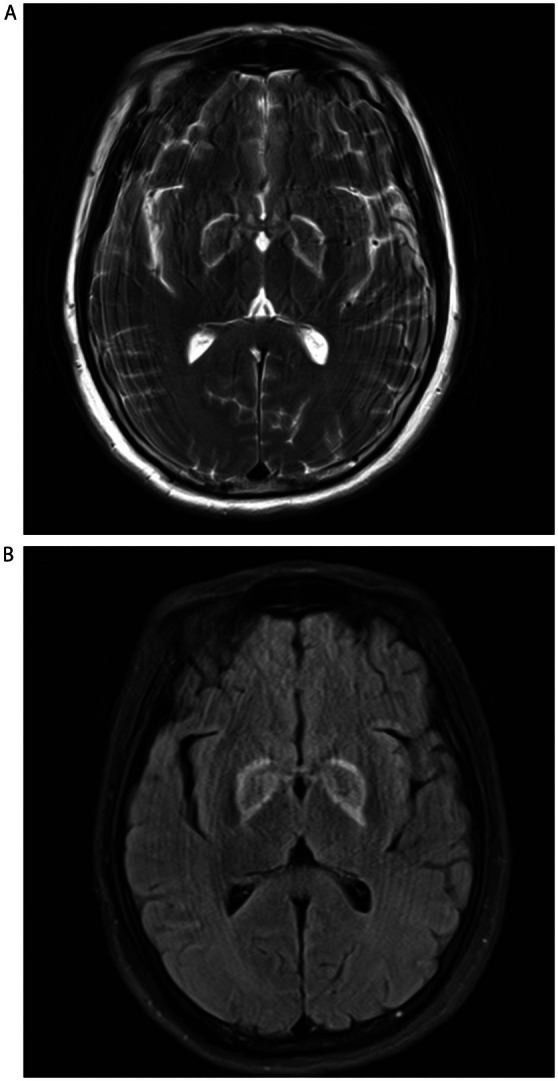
Brain T2‐weighted magnetic resonance imaging demonstrated symmetrical high signal intensity and slightly longer T2 signals in the bilateral basal ganglia, corpus callosum body and periventricular, and centrum semiovale (Figure 2A); the diffusion‐weighted imaging sequences revealed specific symmetrically higher signal lesions in the bilateral basal ganglia, corpus callosum, cerebral cortex, periventricular, centrum semiovale and hippocampus (Figure 2B).
